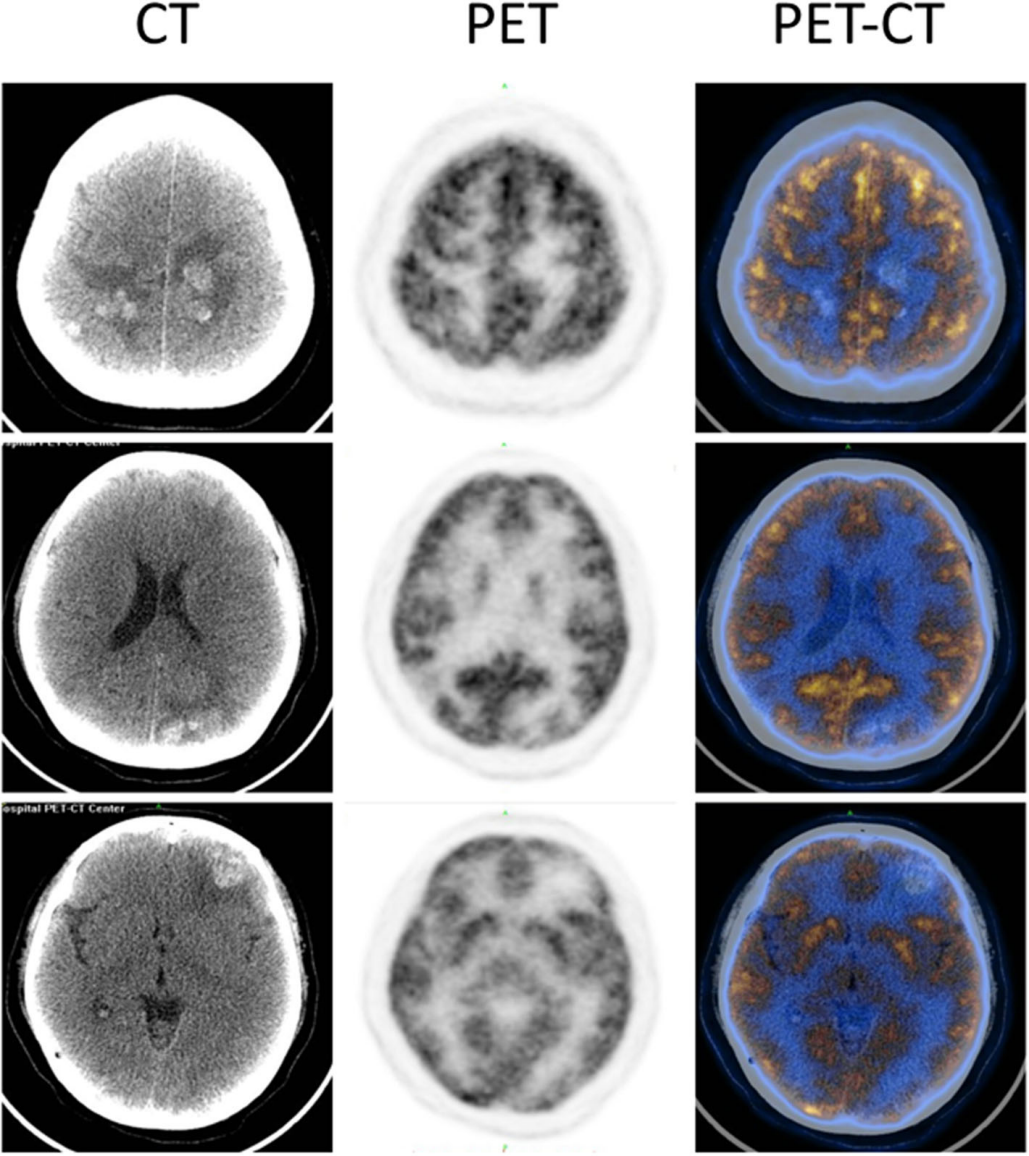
^18^F-FDG PET images show no obvious FDG uptake in the lesions of brain
